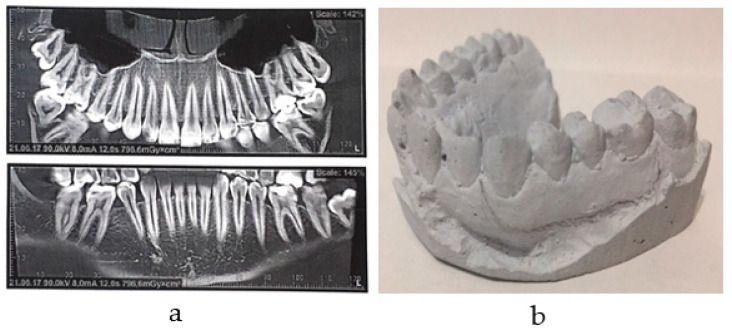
Geometry of the clinical cases: (a) CT image; (b) the gypsum model of an upper jaw.
